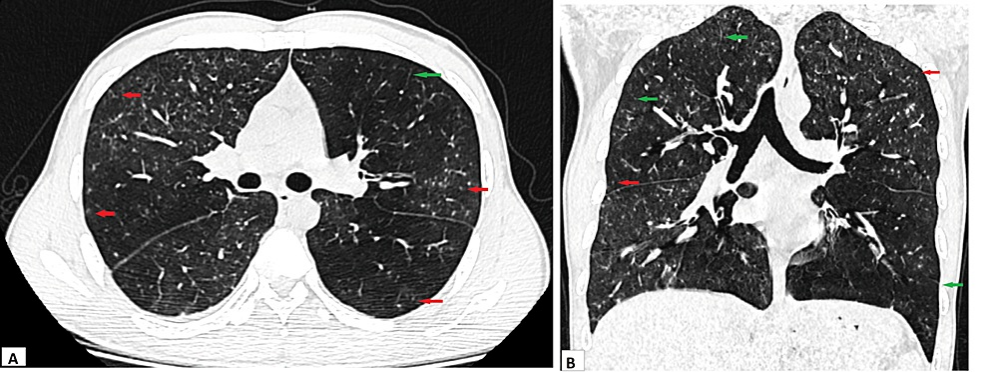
HRCT scan of thorax axial view (A) and coronal view (B) showing diffuse centrilobular nodules (red arrows) and smooth interlobular septal thickening (green arrows) along with mosaic attenuation in the bilateral lung parenchyma. HRCT: High-resolution computed tomography Red arrows indicate centrilobular nodules. Green arrows indicate smooth interlobular septal thickening.
